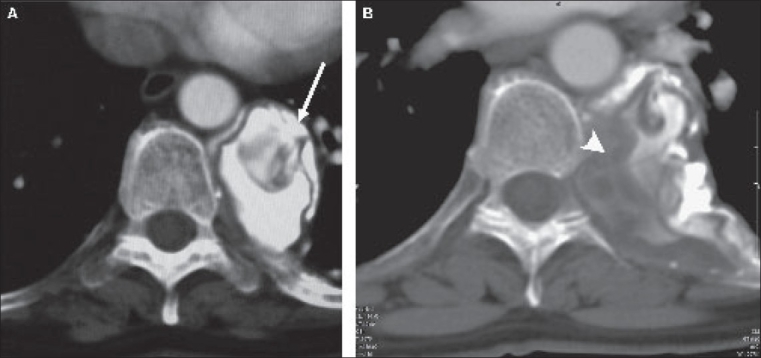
Axial contrast-enhanced CT of the chest showing a predominantly calcified mass in the left paravertebral region (arrow in A) with a noncalcified portion showing a few thin septae (arrowheads in B) representing daughter cysts
